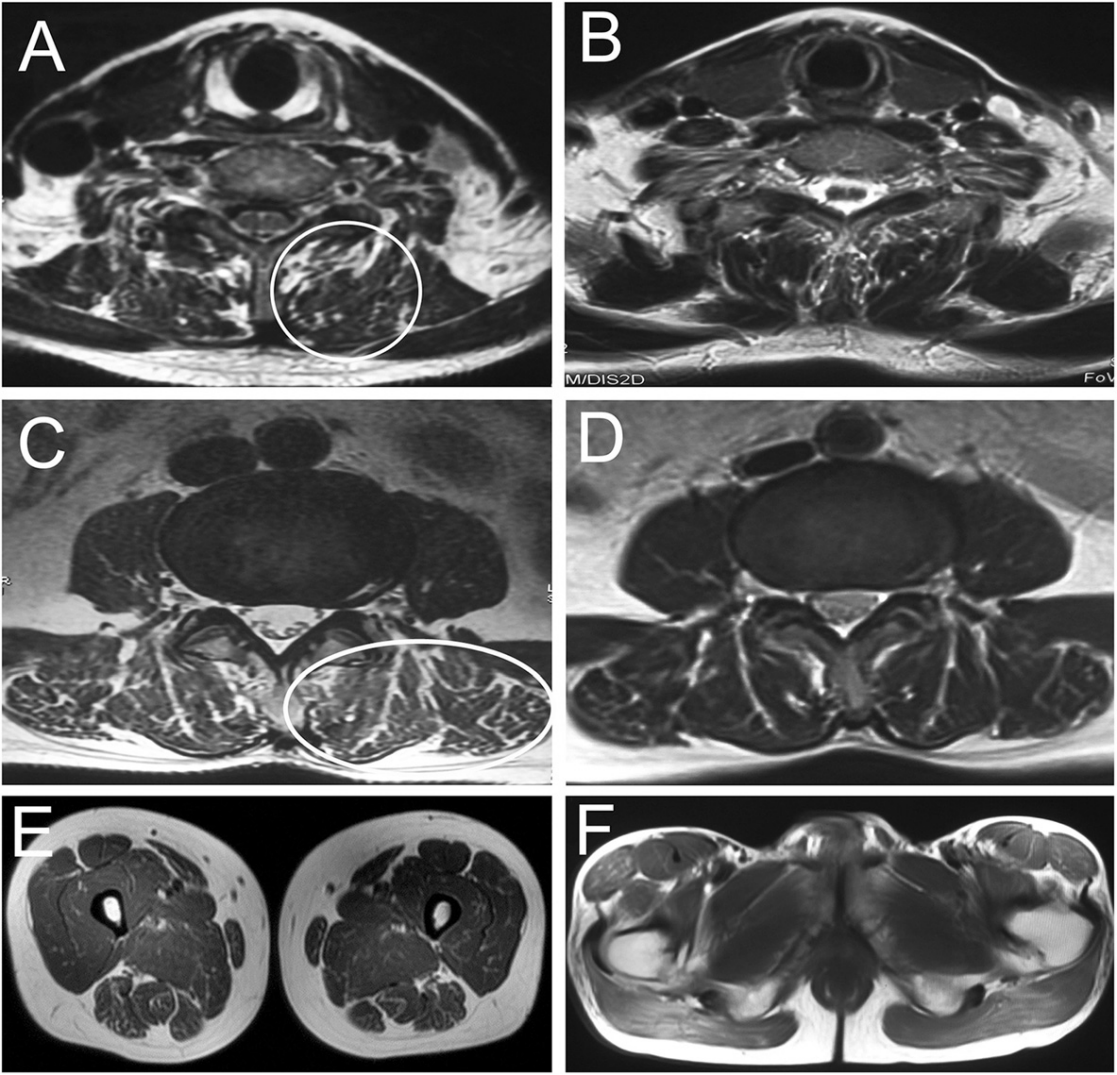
Dynamic changes of muscular MRI. Cervical MRI revealed a few abnormal signals infiltrating into cervical paravertebral muscles in T2 weighted image (a, circle), while the abnormal signals almost disappeared after riboflavin treatment (b). Lumbosacral MRI showed a lot of abnormal signals diffusing around musculi multifidus, musculi longissimus, and iliopsoas in T2 weighted images (c, ellipse), while the abnormal signals almost disappeared after riboflavin treatment (d). Lower limb MRI showed normal pattern of musculi biceps femoris, musculi quadriceps femoris (e), and gluteus maximus (f)
